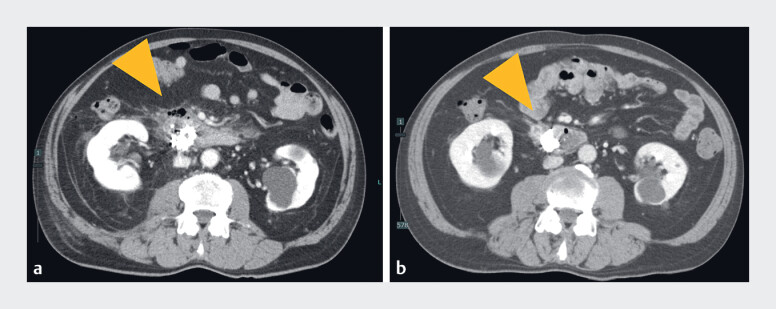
Contrast-enhanced abdominal computed tomography after endoscopic submucosal dissection (ESD) showing abscess (arrowhead).
